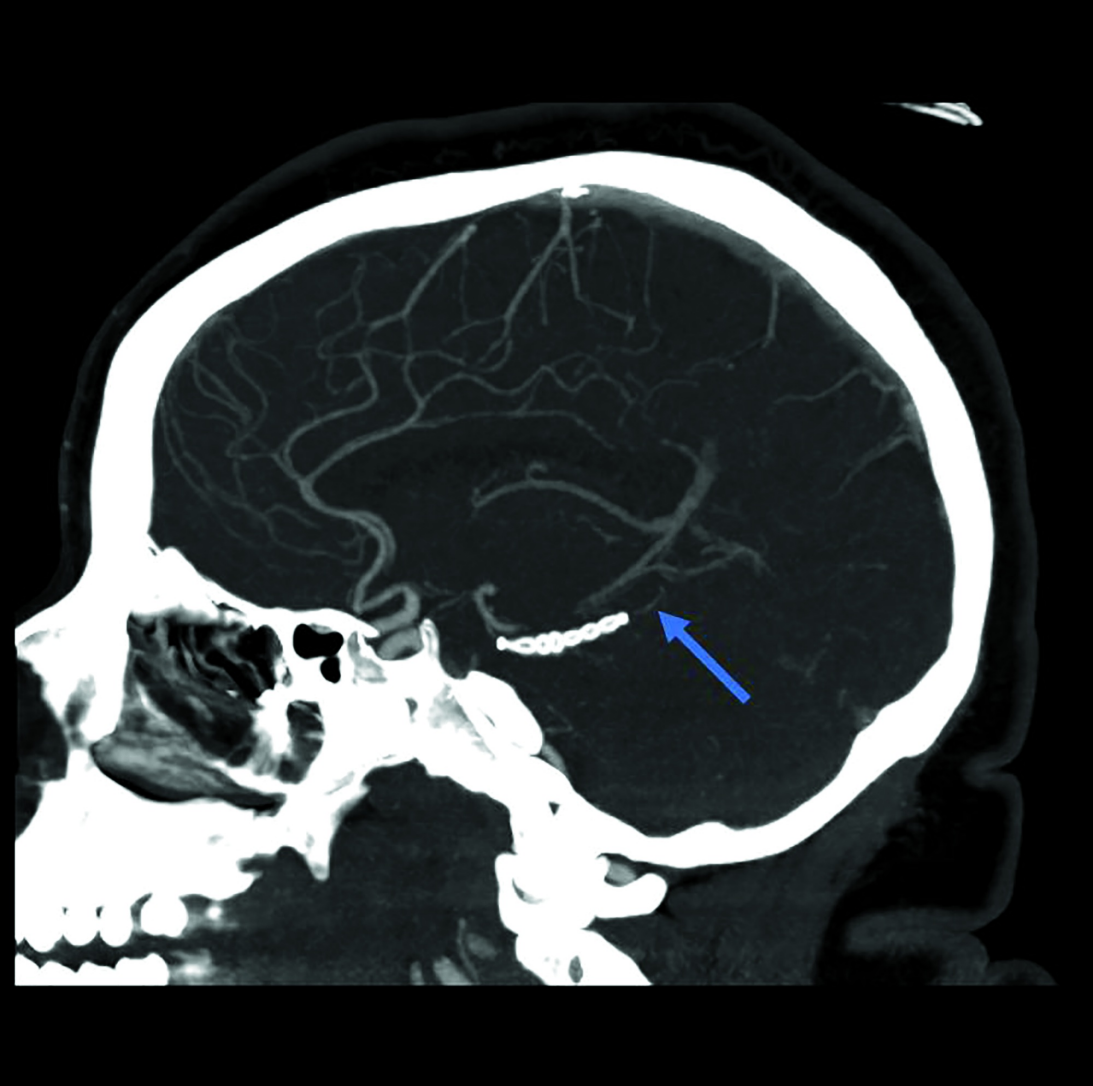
Computed tomography angiogram Computed tomography angiogram at the 3-month follow-up demonstrating the left superior cerebellar artery low-profile visualized intraluminal support Jr. stent with preserved flow both proximal and distal to stent (blue arrow).
